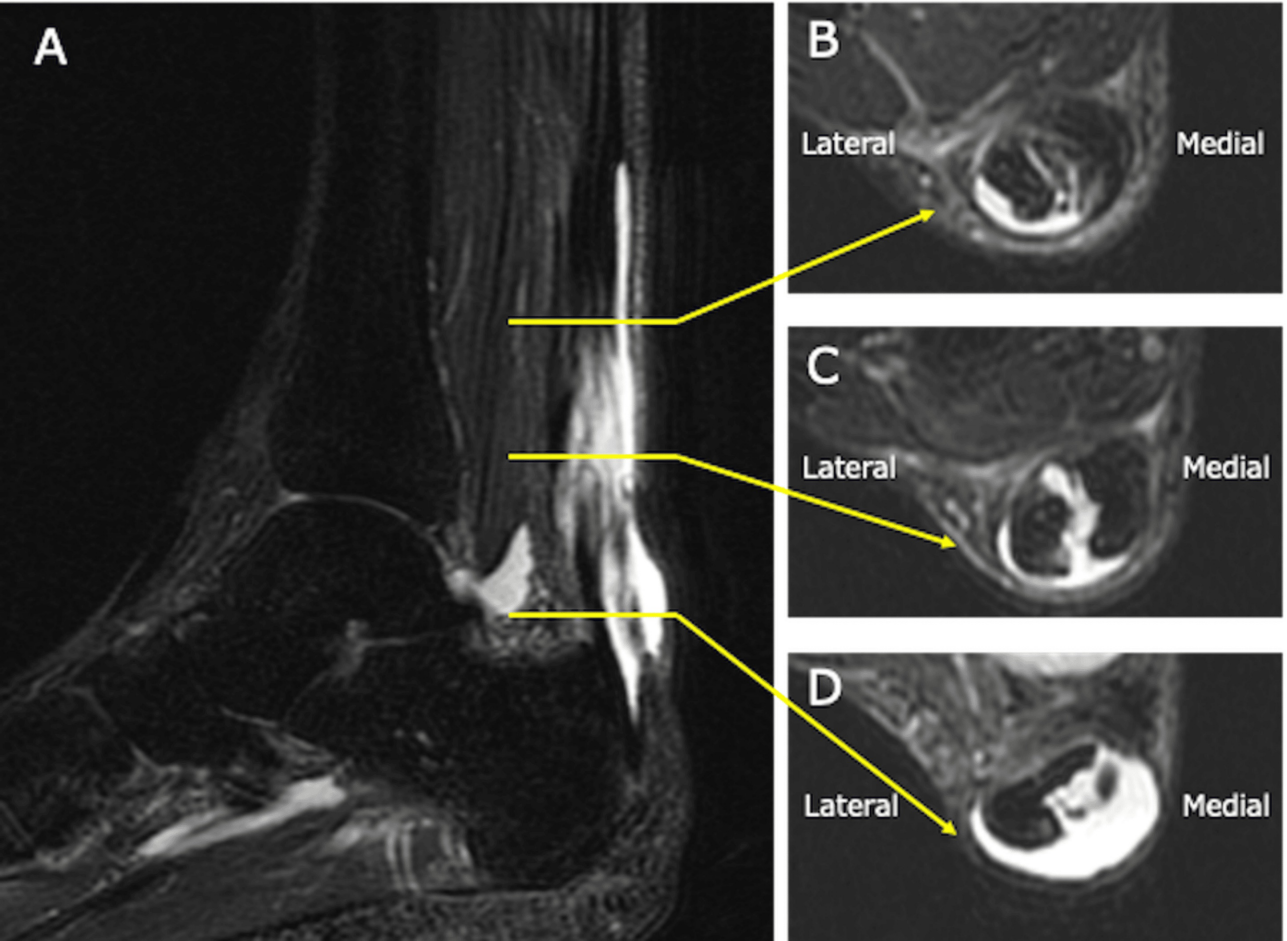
Preoperative magnetic resonance imaging The Achilles tendon showed a split tear involving approximately 50% of the tendon width from the medial side, extending from the proximal portion to the calcaneal insertion, with absence of the medial tendon fibers at the insertion site. A: sagittal view of the Achilles tendon; B: axial view of the proximal Achilles tendon; C: axial view of the mid portion of the Achilles tendon; D: axial view of the proximal Achilles tendon
